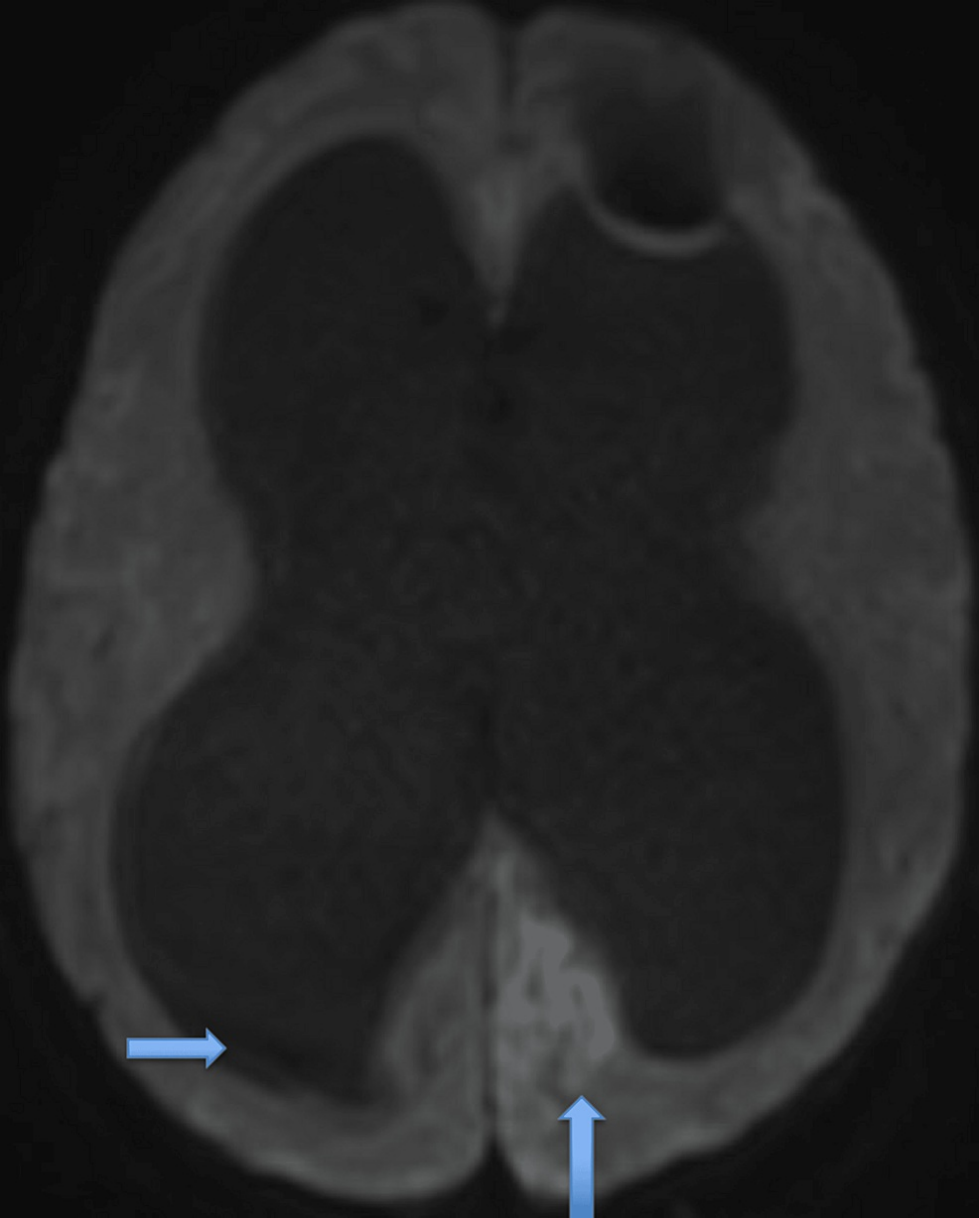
Magnetic resonance imaging of the brain showing multiple acute infarcts in the bilateral thalami and left posterior cerebral artery distribution (upward arrow). Also shows marked ventriculomegaly with trans-ependymal edema (side arrow).
